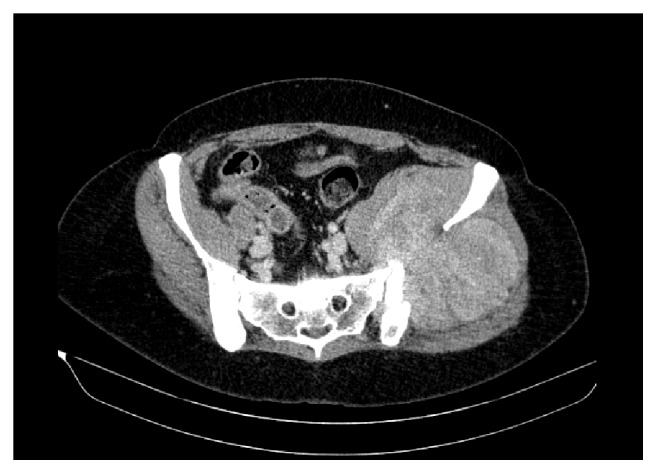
Contrast-enhanced CT. Axial view. There is a heterogeneous enhancing mass destroying the left iliac bone and expanding both in and out of the pelvic cavity. The iliac vessels have deviated towards the right. No evident infiltration of pelvic structures was observed.
